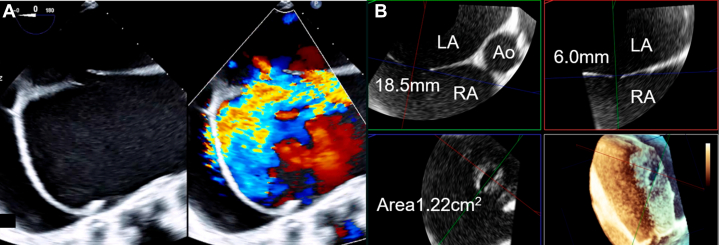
Rapid Morphological Evolution Emergency transesophageal echocardiography during the acute phase. (A) Side-by-side 2-dimensional B-mode and color Doppler views capturing the high-velocity tricuspid regurgitation jet surging directly into the iASD, acting as a “hydrodynamic wedge.” (B) Repeat 3-dimensional quantification analysis documenting a dramatic expansion of the iASD; the orifice area more than doubled to 1.22 cm^2^ (major/minor axis: 18.5/6.0 mm). This rapid evolution underscores the mechanical fragility of the remodeled septum under the mechanical stress of high-kinetic eccentric jet impingement. iASD = iatrogenic atrial septal defect; LA = left atrium; RA = right atrium.
